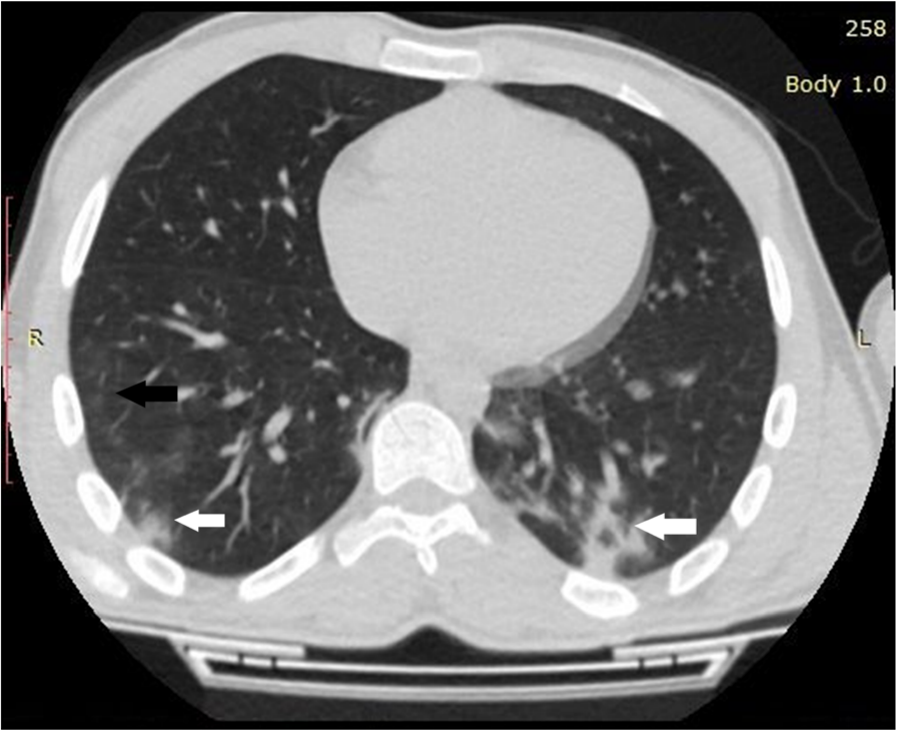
Axial non-contrast enhanced CT chest of 16-year-old girl, 2 days after the onset of fever, there are patches of ground glass opacities (black arrow) with consolidation (white arrow) distributed peripherally in the posterior segments of both lower lung lobes
